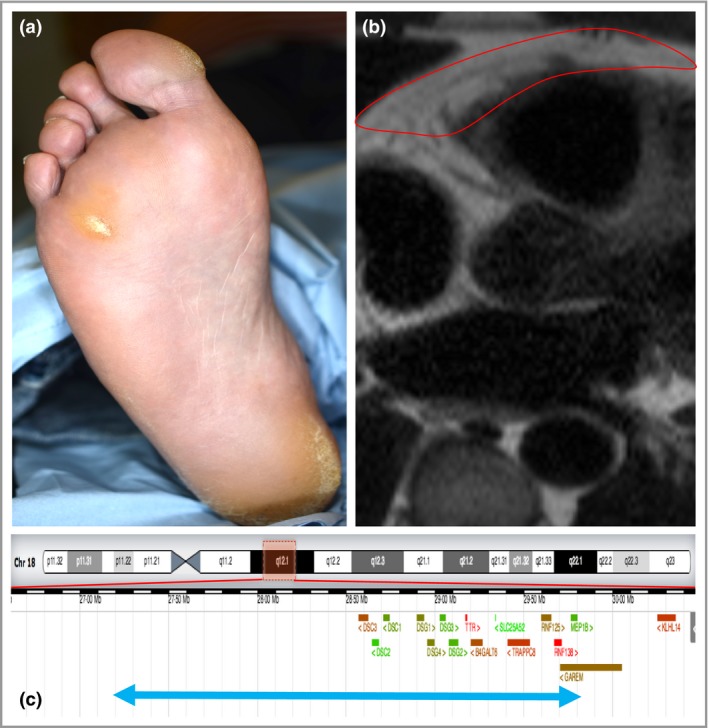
(a) Focal keratoderma predominantly over pressure areas and regions in contact with footwear. (b) Cardiac magnetic resonance imaging with the right ventrical outlined in red, where fibrofatty change is noted as a high‐signal region. (c) Span of the 2·6‐Mb deletion indicated by the blue arrow, with genes indicated in red and green.
